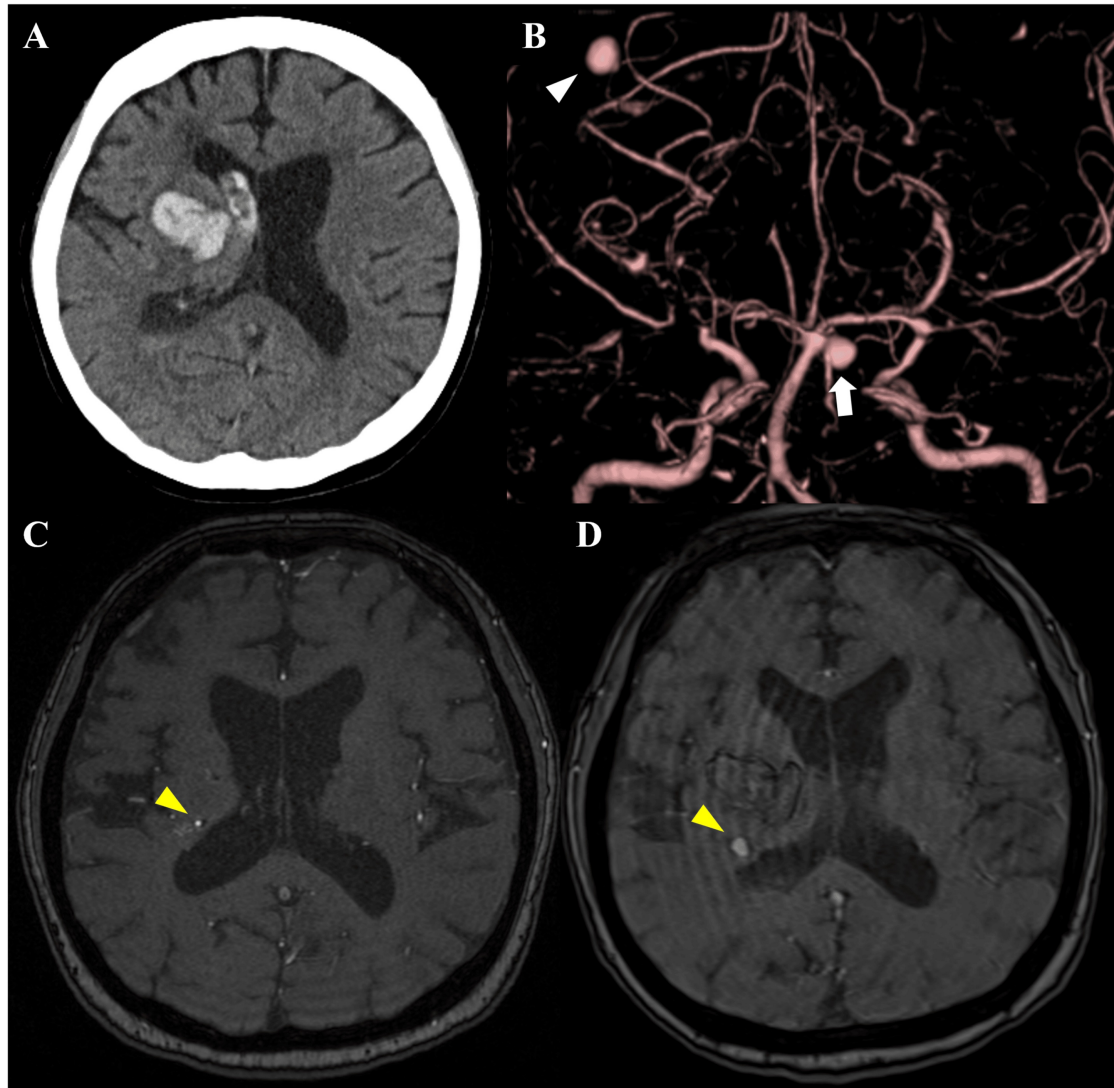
Radiographic images on admission. A head computed tomography (CT) scan in the emergency room revealed a right thalamic and intraventricular hemorrhage (A). Three-dimensional CT angiography identified two aneurysms: one in the left basilar-superior cerebellar artery (white arrow) and another in the right cerebral hemisphere behind the hematoma (white arrowhead) (B). A magnetic resonance angiography (MRA) performed two months prior to her presentation showed a small aneurysm of about 1 mm in the right corona radiata (C), and an MRA performed the day after presentation disclosed the aneurysm had grown to approximately 5 mm (yellow arrowheads) (D).
